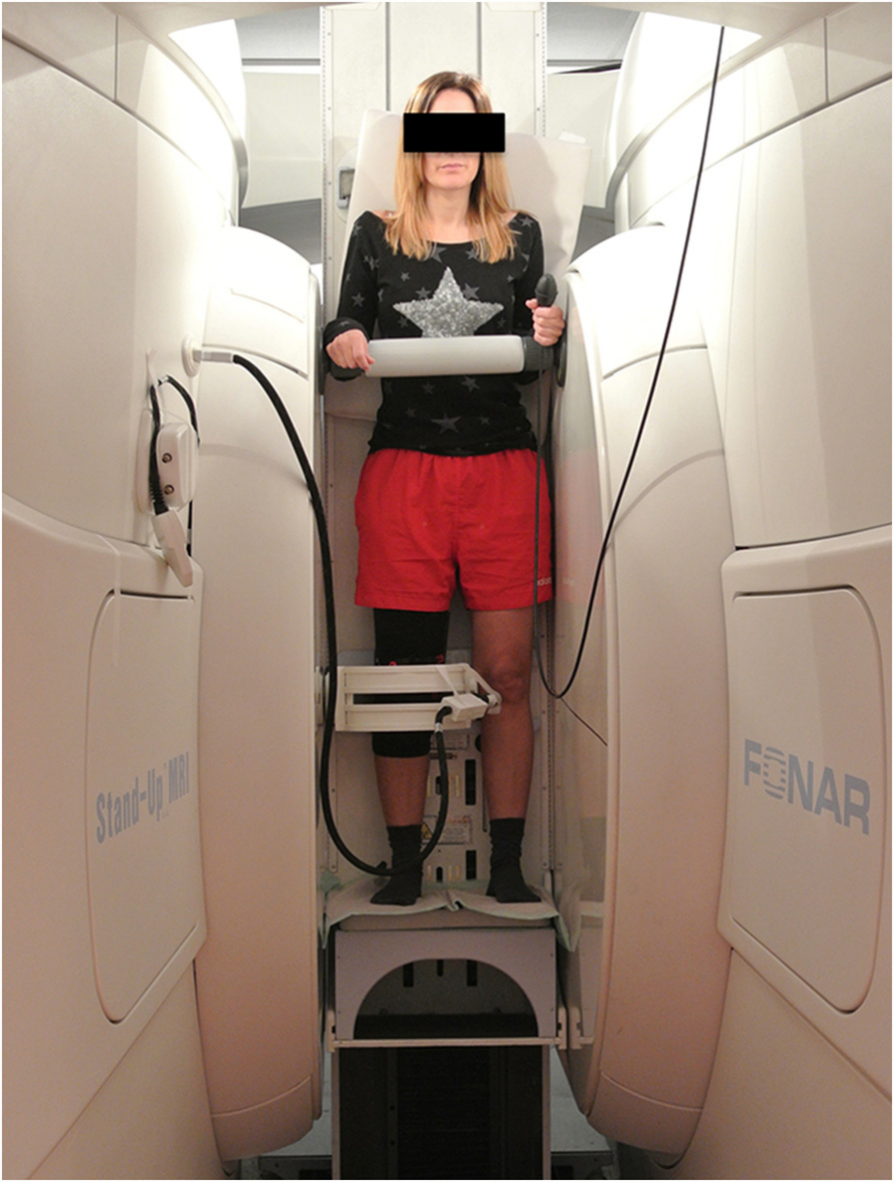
The upright open-MRI scanner with a subject examined at full extension weight-bearing condition
